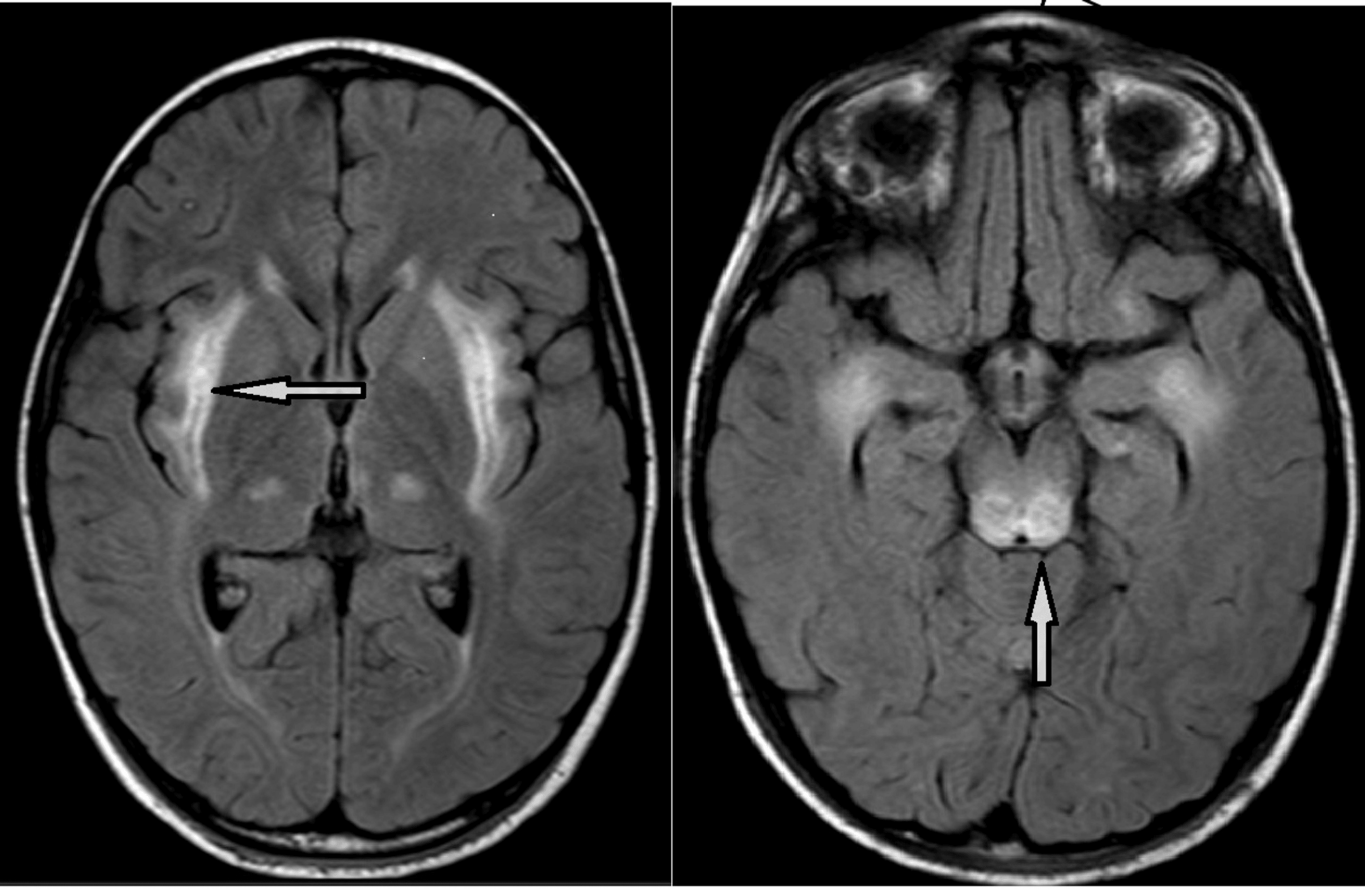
FLAIR axial images showing hyperintense signals in the external capsules, both thalami, dorsal pons, and hippocampi, with relative sparing of the basal ganglia. The arrow in the left-side MRI image indicates a hyperintense signal in the external capsule, while the arrow in the right-side image points to the signal in the dorsal pons. FLAIR: Fluid-attenuated inversion recovery
